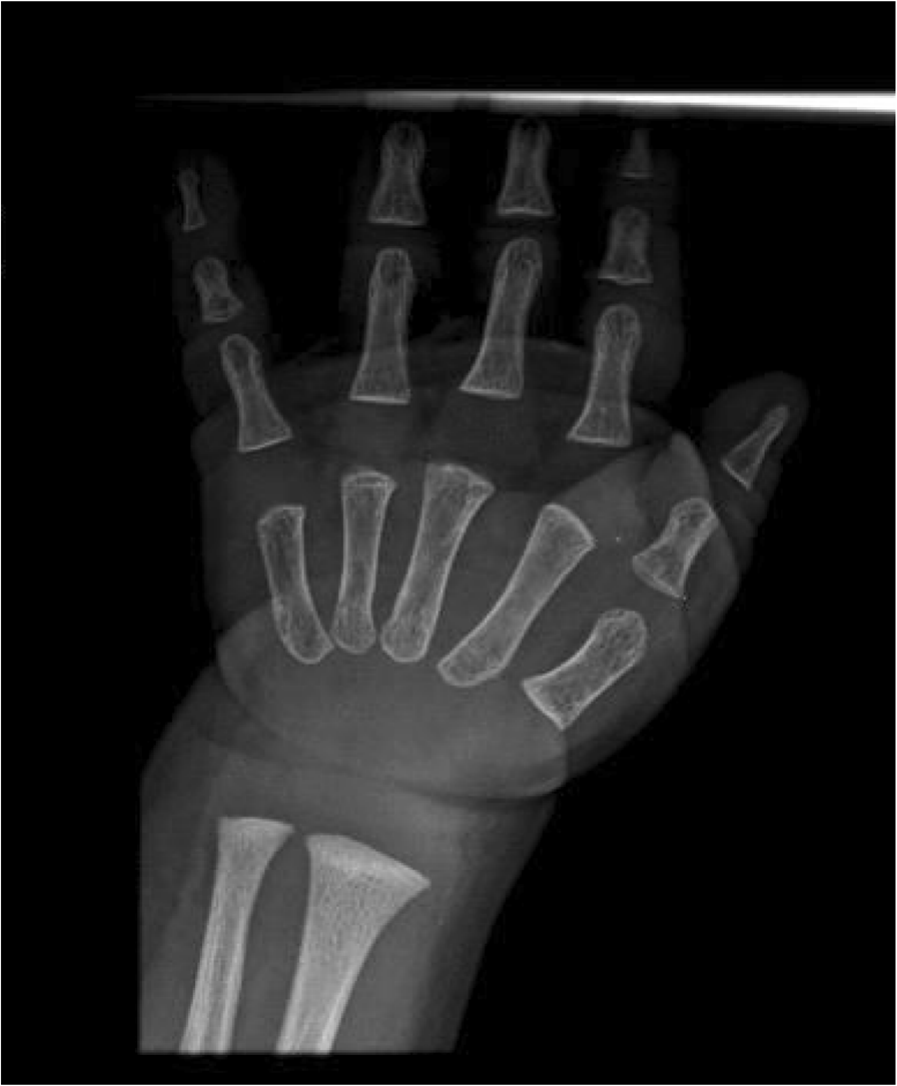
Radiograph of patient’s left hand. Delayed bone maturation, cone-shape appearance of distal phalanges. (We could not perform a morphologic radiographic study due to the lack of images for third and fourth distal phalanges)
